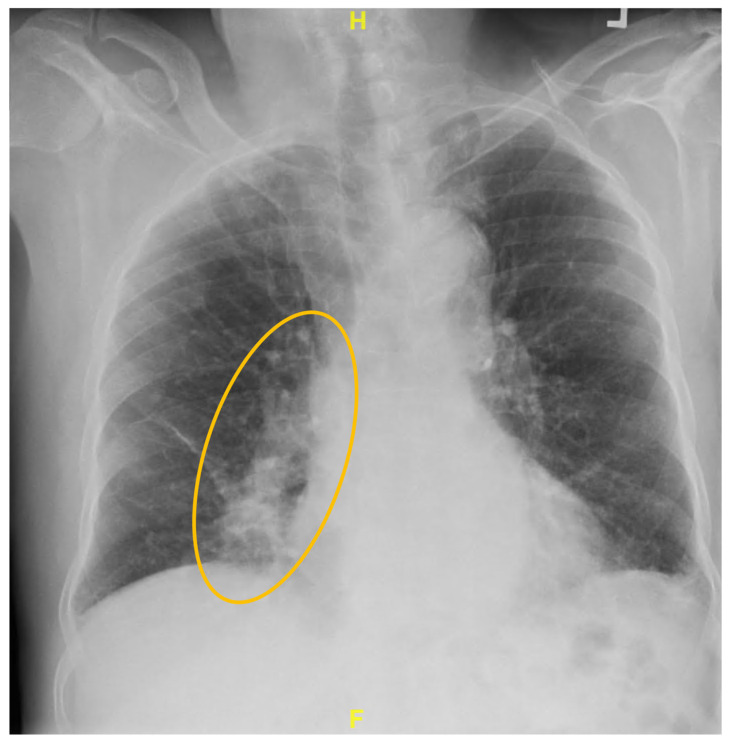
Chest Xray (PA view): Yellow circle demonstrating fibronodular infiltrates in the right middle lobe.
